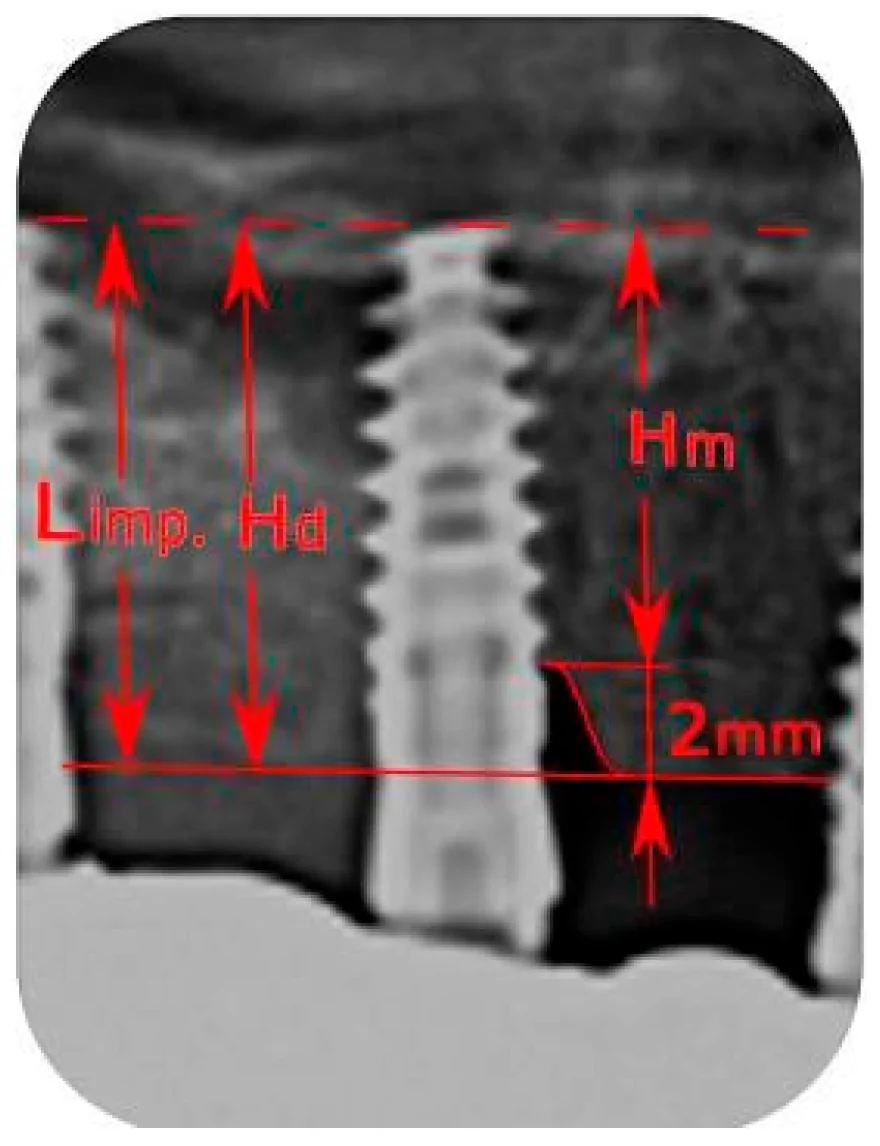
Representative standardized periapical radiograph used for marginal bone-level assessment around a dental implant. Implant length (Limp) and mesial and distal bone height measurements (Hm and Hd) were used to calculate marginal bone resorption relative to the implant–abutment reference level. Measurements were performed on calibrated digital radiographs obtained using the paralleling technique.
